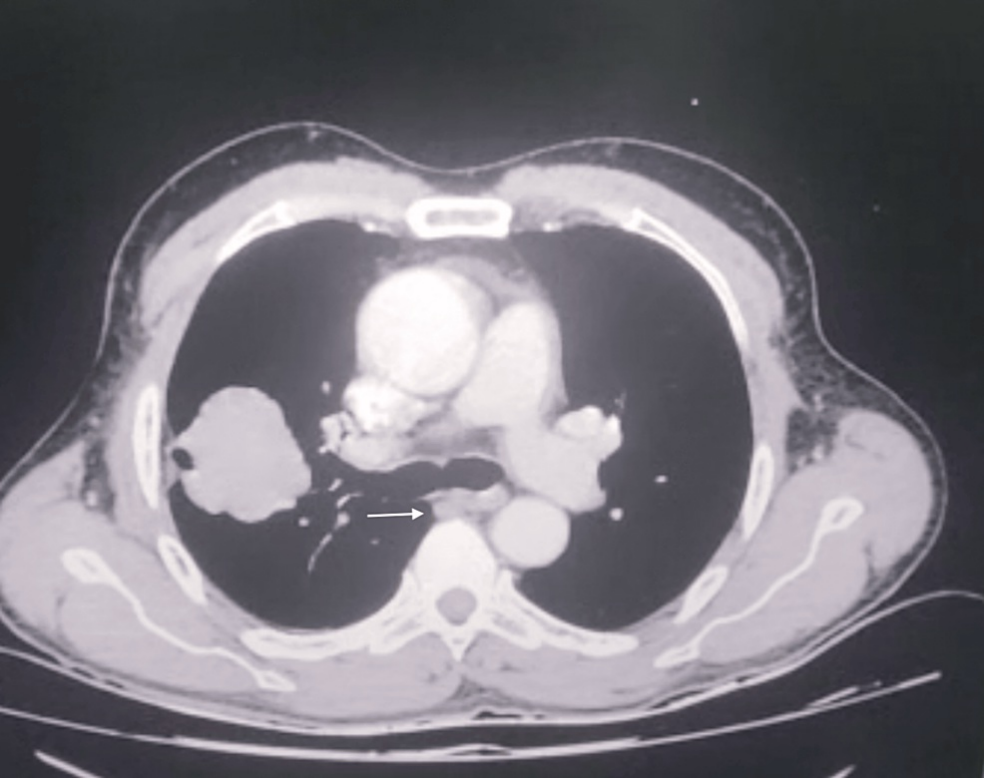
Chest HRCT (mediastinal window)
